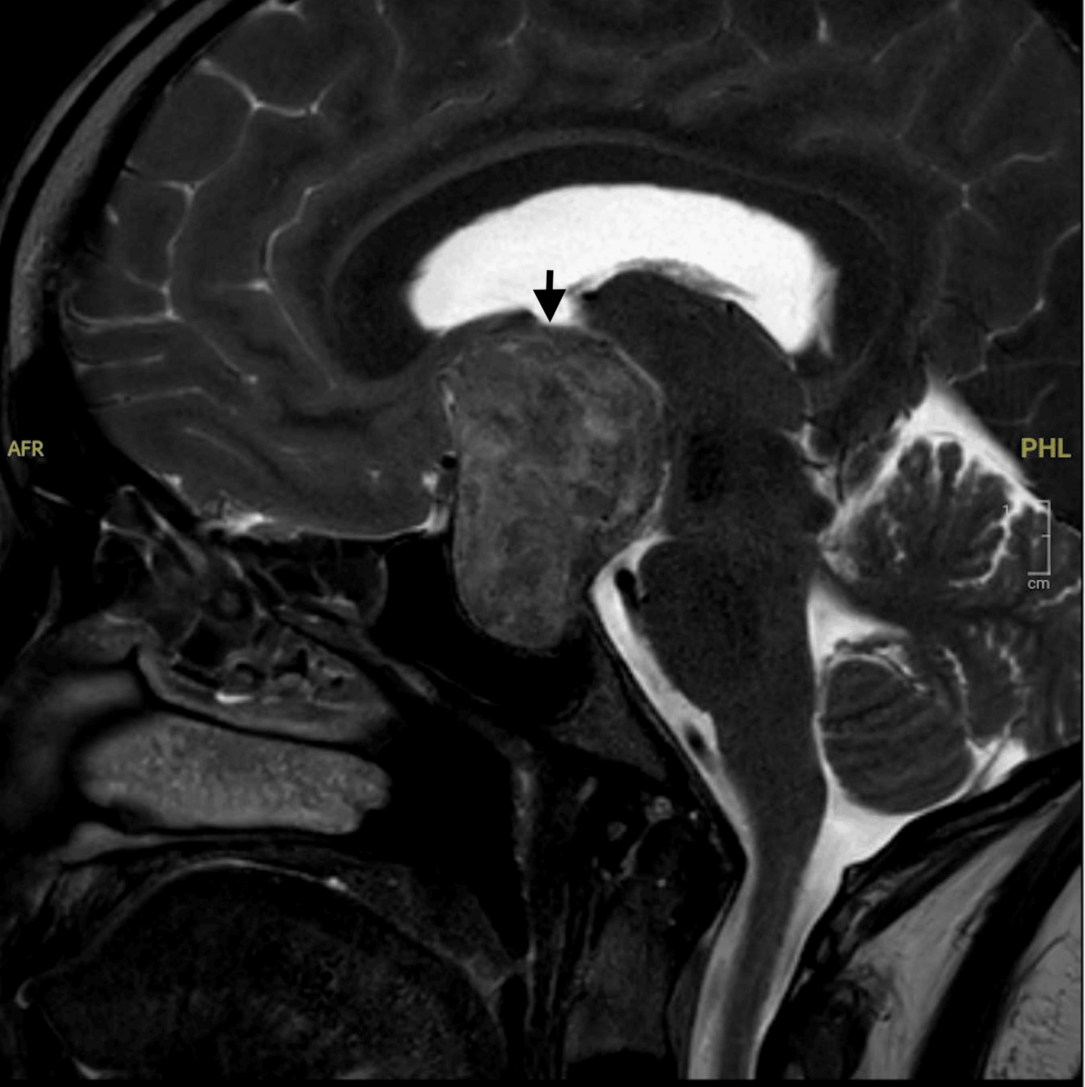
T2-weighted sagittal section of the brain showing a large solid, heterogenous sellar suprasellar mass lesion (black arrow) measuring about 34 x 32 mm (transverse and anteroposterior). The pituitary gland is not seen separately
